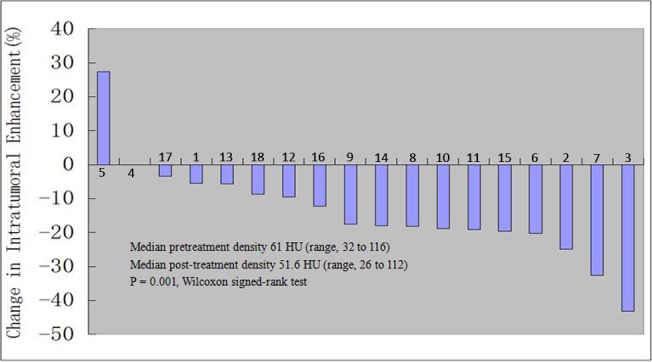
Change of the average tumor CT value in the 18 patients.
